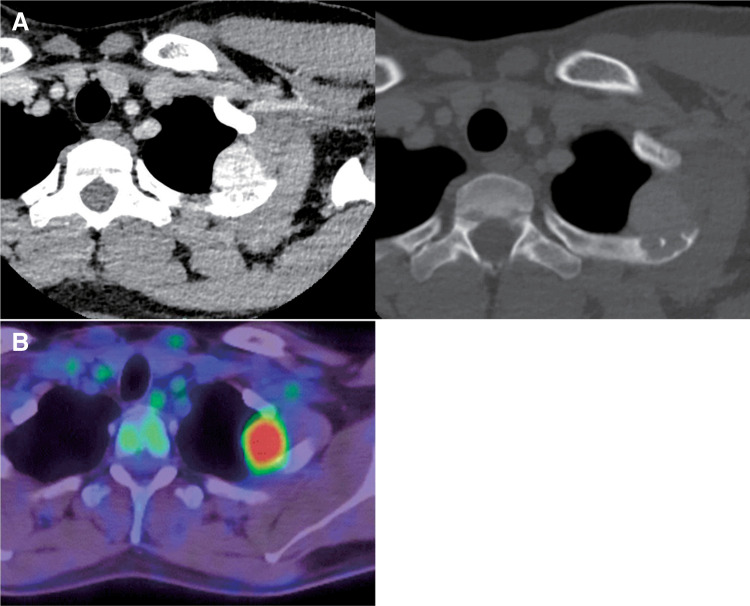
(A) Chest CT visualized a 2.5 × 2.0 cm nodule with a clear border spreading to the left first intercostal muscle and second rib. The nodule showed contrast enhancement. (B) PET revealed FDG accumulation in the nodule, with a maximum SUV of 8.48. CT, computed tomography; PET, positron emission tomography; FDG, fluorodeoxyglucose; SUV, standardized uptake value
